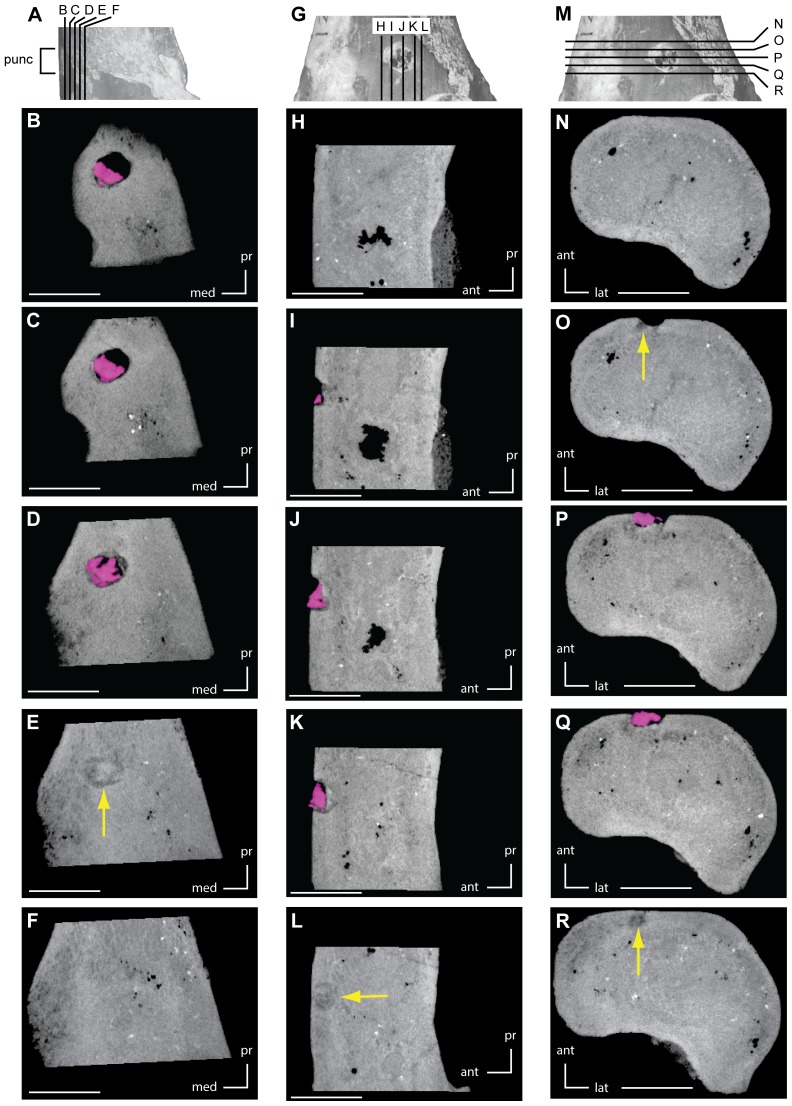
CT images of embedded tooth fragment and associated puncture on right femur (UMNH VP 21107). A. Right femur in medial view showing orientation of images B–F. B–F. CT images taken along the coronal plane, arranged in order from anterior to posterior. G. Right femur in anterior view showing orientation of images H–L. H–L. CT images taken along the sagittal plane, arranged in order from lateral to medial. M. Right femur in anterior view showing orientation of images N–R. N–R. CT images taken along the transverse plane, arranged in order from proximal to distal. Pink areas outline the embedded tooth fragment, while yellow areas indicate regions of compression damage to the surrounding bone. Scale bars equal 5 mm. Abbreviations: ant, anterior; lat, lateral; med, medial; pr, proximal; punc, puncture.
